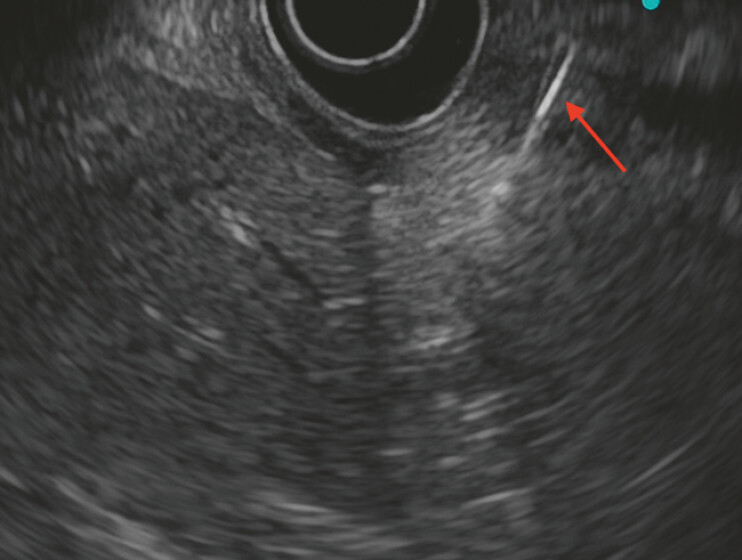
Endoscopic ultrasound shows the location and depth of the fishbone.
